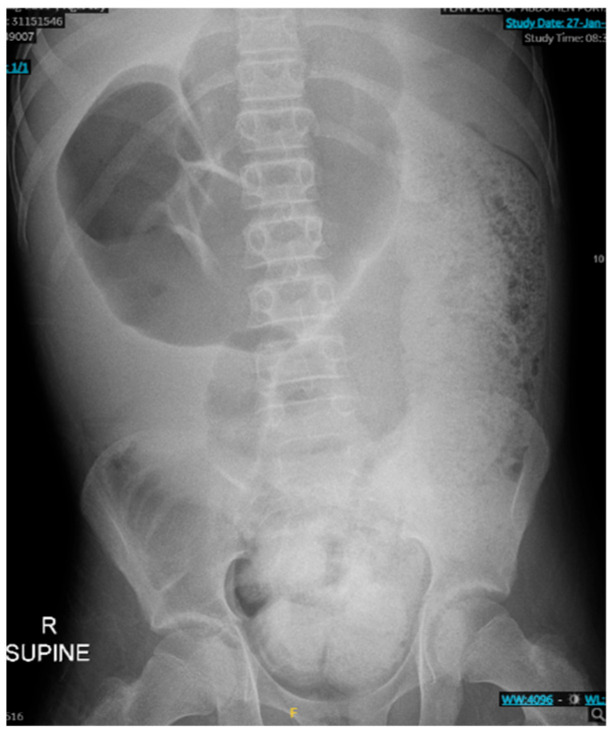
CT scan on patient presentation, revealing a colon dilated to 6.34 cm in diameter, with significant stool burden.
